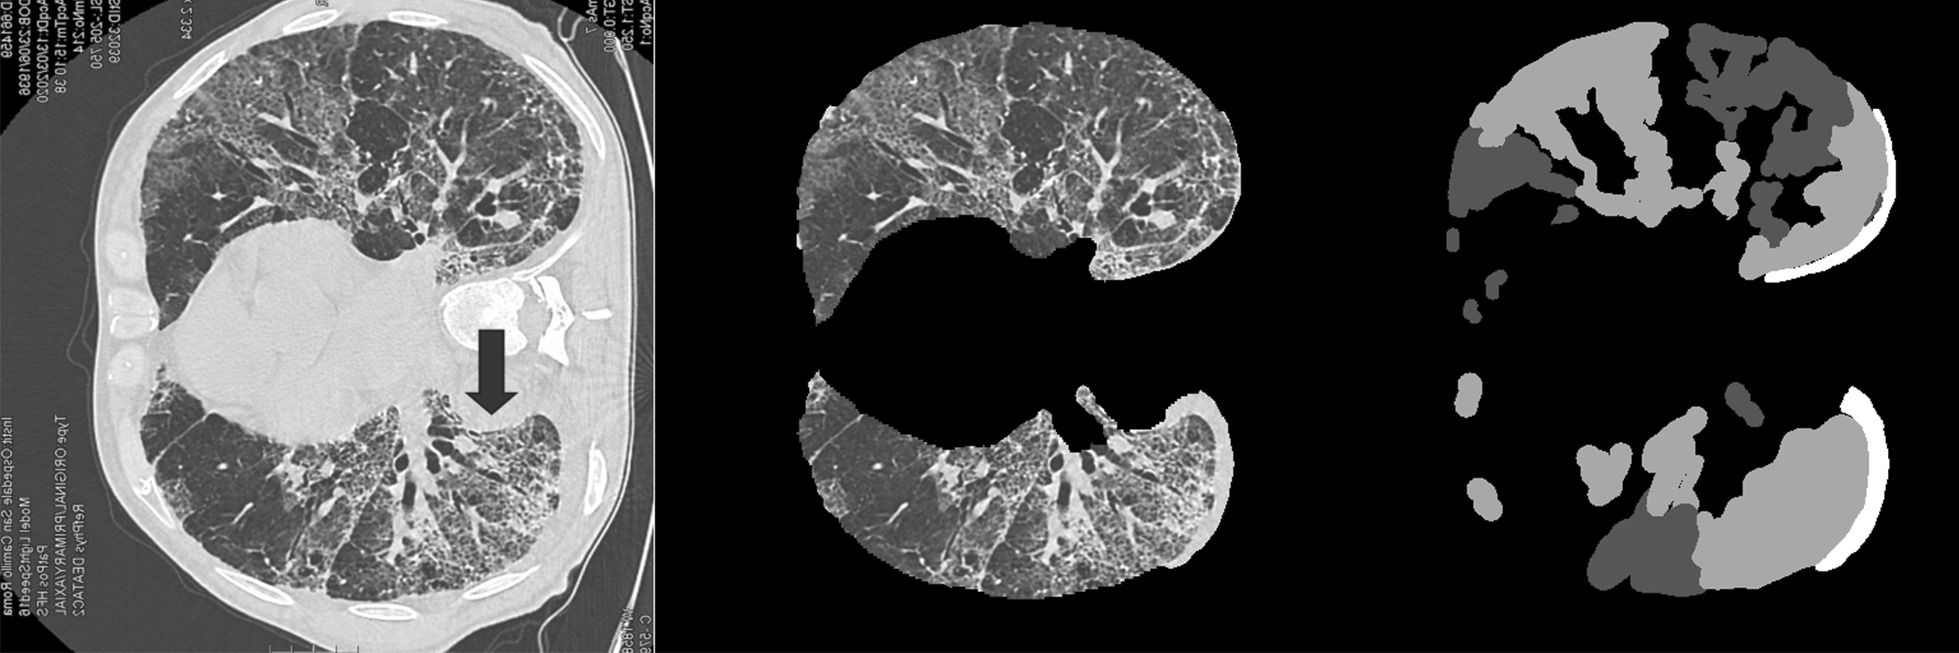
Dataset sample. CT scan (left), masked lungs (middle), and labeled classes (right), where black is class C_0_, dark gray is C_1_, light gray is C_2_, and white is C_3_.
